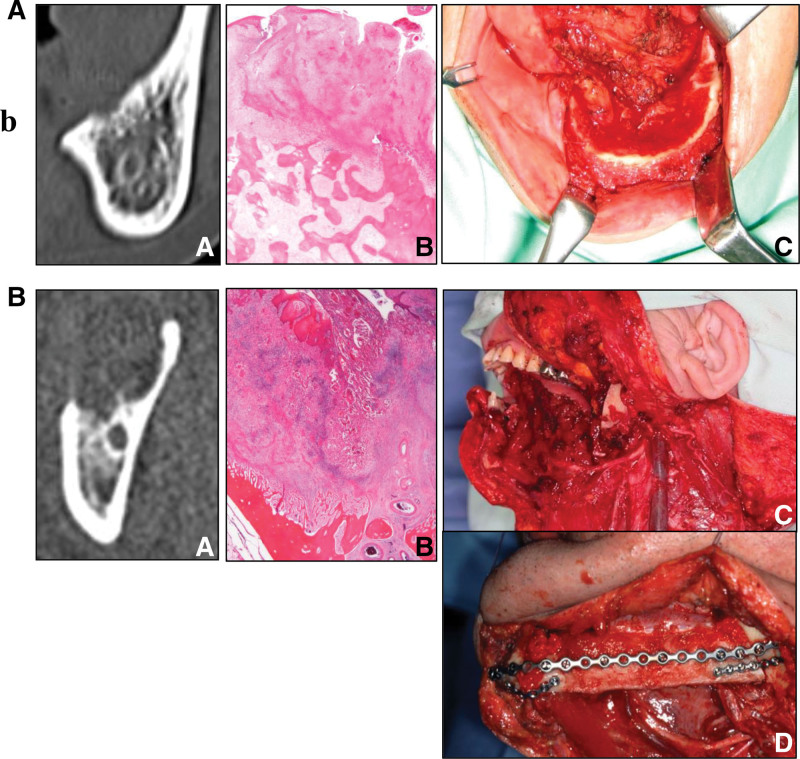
Vertical mandibular invasion and operation methods of lower gingival squamous cell carcinoma. If no mandibular invasion is diagnosed, bone scraping or corticotomy is performed to achieve safe margins. Marginal mandibular resection is required for cortical bone invasion alone, and segmental mandibular resection if bone marrow invasion is diagnosed. Patients undergoing segmental mandibular resection requires reconstructive surgery. (A) Invasion limited to the cortical bone: a) CT imaging, b) pathological image, and c) marginal mandibular resection. (B) Invasion of the bone marrow: a) CT imaging, b) pathological image, c) segmental mandibular resection, and d) oromandibular reconstruction with vascularized free fibular flap. CT = computed tomography.
